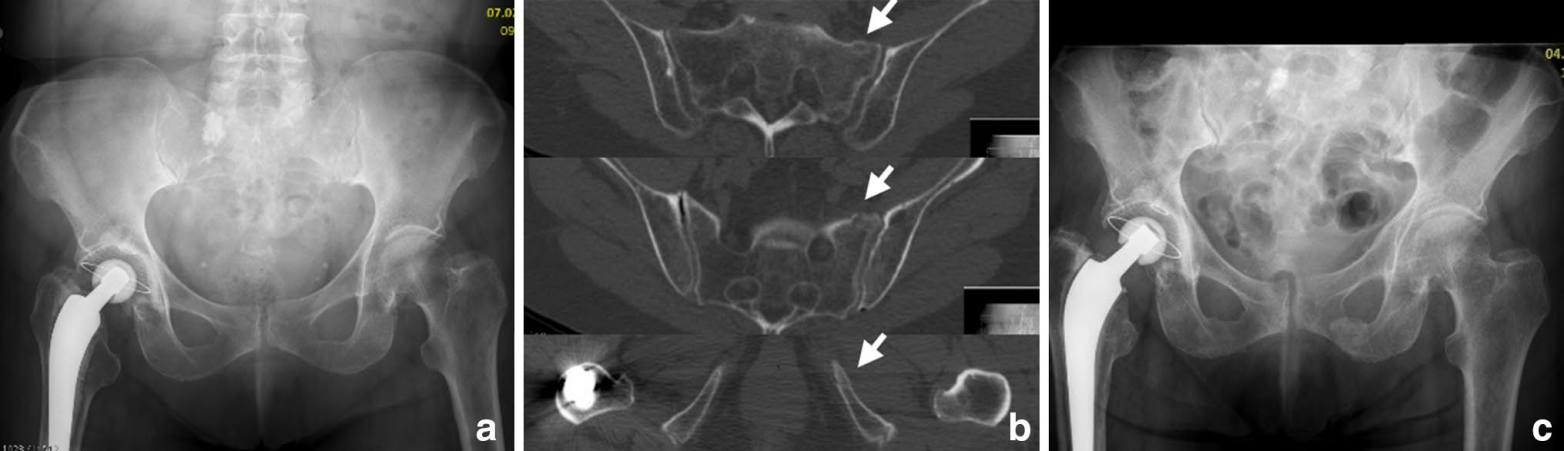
FFP type IIb: 81-year-old female with a crush injury of the left sacral ala and a non-displaced fracture of the left anterior pelvic ring (b). With conservative management she went on to consolidation (c radiograph 13 months after trauma)
